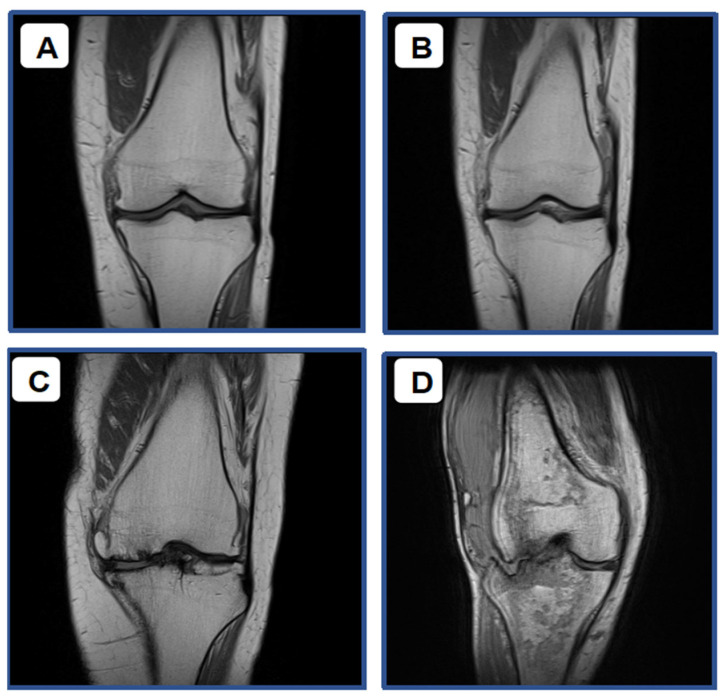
Degree of Articular Cartilage Degradation in Knee OA based on MRI (Outerbridge Score): (A). Grade 1; (B). Grade 2; (C). Grade 3; (D). Grade 4.
